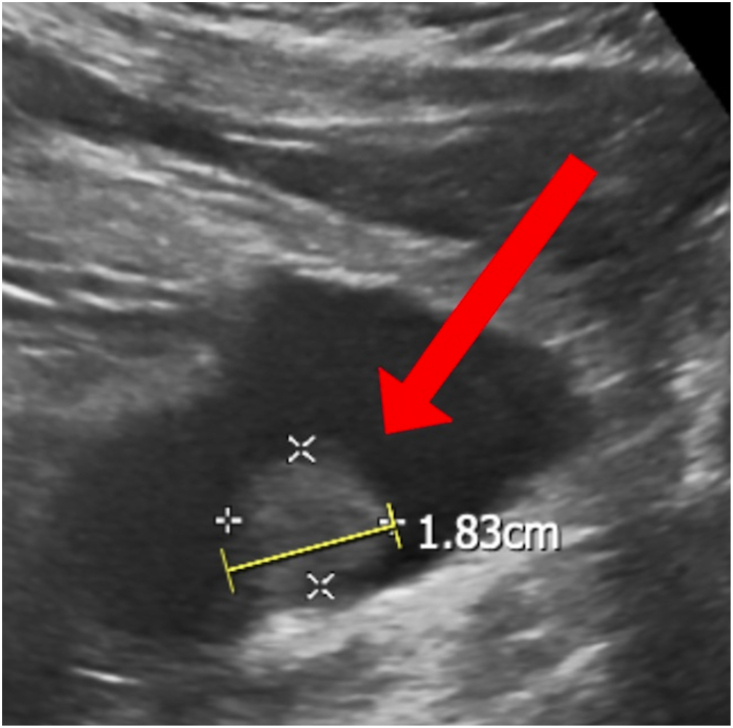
Bladder ultrasound transverse view obtained two years after prostate aquablation demonstrating a new mass along the right lateral bladder wall measuring approximately 1.8 cm in maximal diameter.
